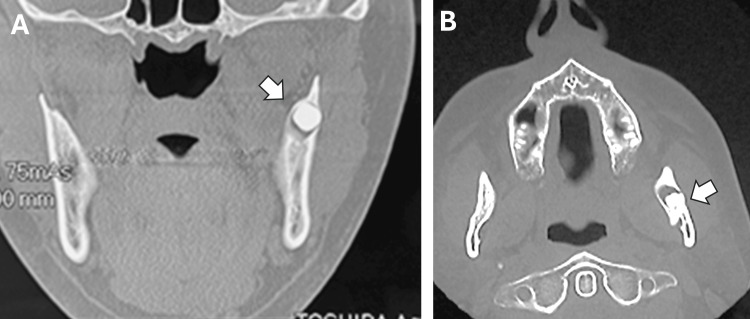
Computed tomography with coronal, sagittal, and axial slices. (A) Coronal and (B) axial CT scans showing a well-defined radiolucency around the crown, suggesting the formation of a dentigerous cyst (arrows).
